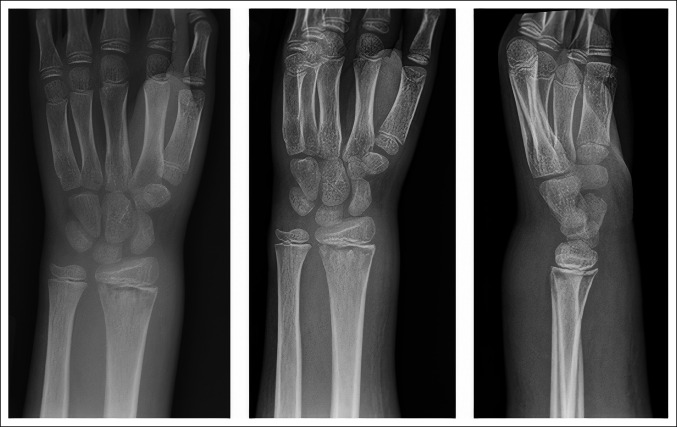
AP, oblique, and lateral radiographs at the time of presentation to our emergency department; diffuse palmar and dorsal soft-tissue swelling noted about the wrist and forearm with mottled appearance of the distal radius physis.
